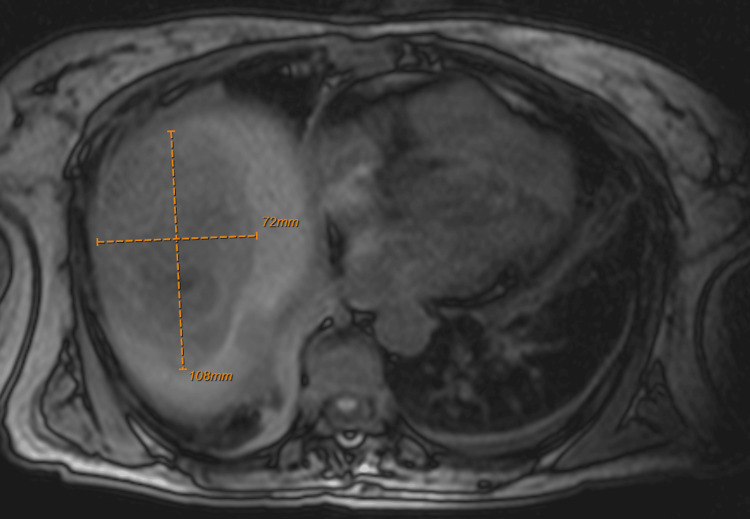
MRI abdomen showing a reduction in the size of the mass
